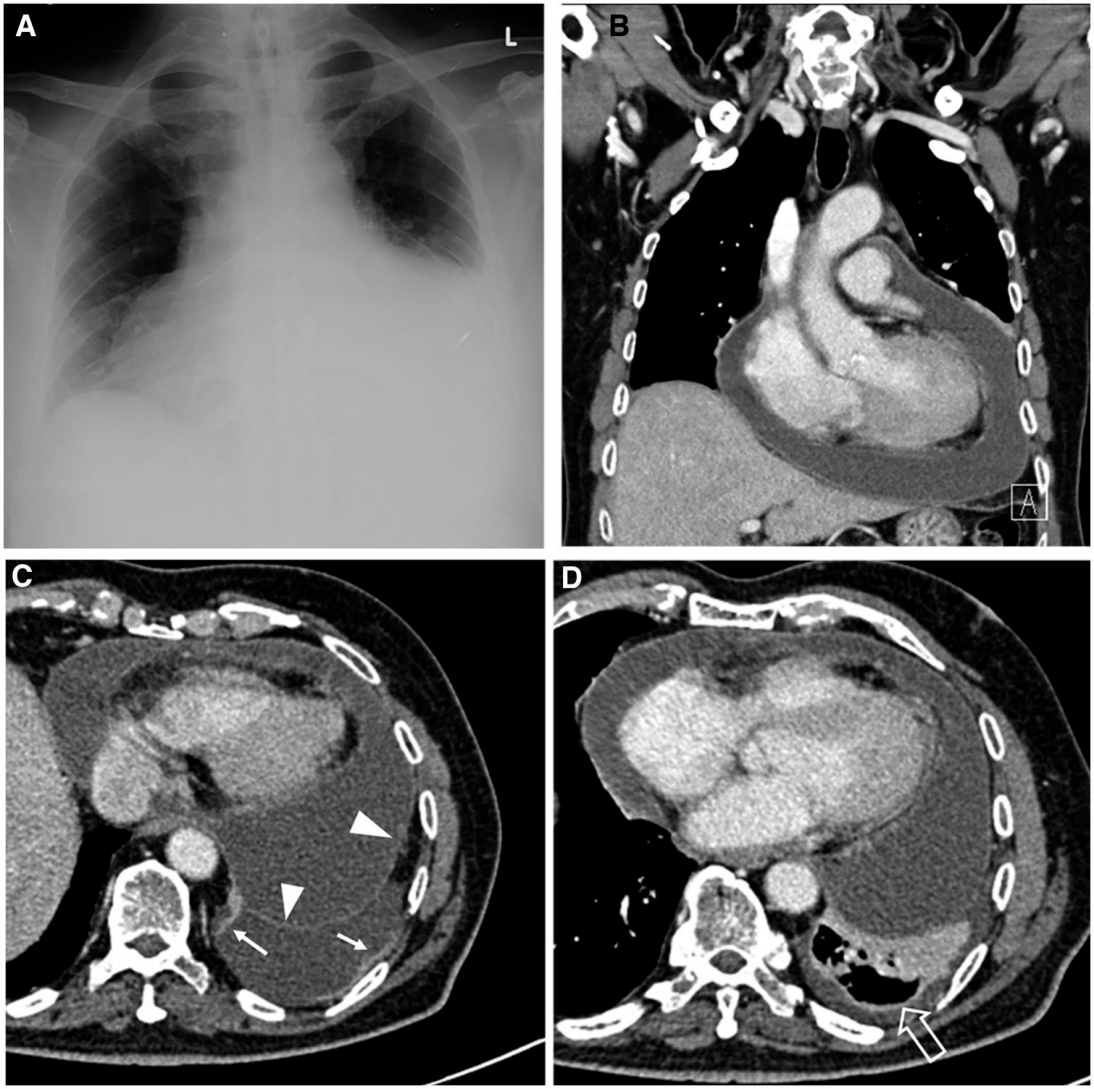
Tuberculous pericarditis and pleuritis in an 81-year-old man. (A) Frontal chest radiograph shows an enlarged globular cardiac shadow with the characteristic “water bottle” appearance. (B) Coronal contrast-enhanced CT image shows pericardial effusion. (C and D) Axial contrast-enhanced CT images show smooth thickening and enhancement of the pericardium (white arrowheads in C), pleural effusion, plaque-like thickening of the parietal pleura (small white arrows in C), thickened visceral pleura (open arrow in D) and compressive atelectasis of the underlying lung.
